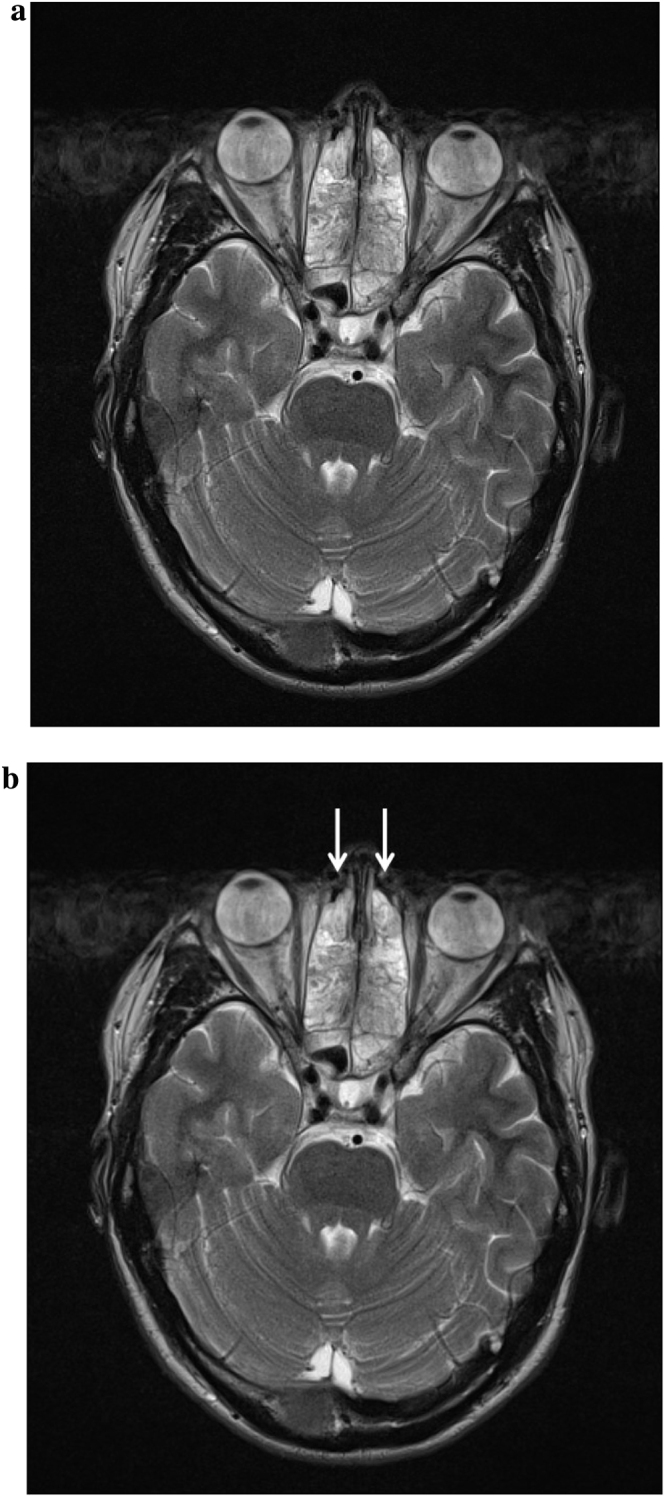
(a) MRI Head (T2 weighted) showing severe ethmoid sinusitis (unmarked). (b) MRI Head (T2 weighted) showing severe ethmoid sinusitis (marked).
